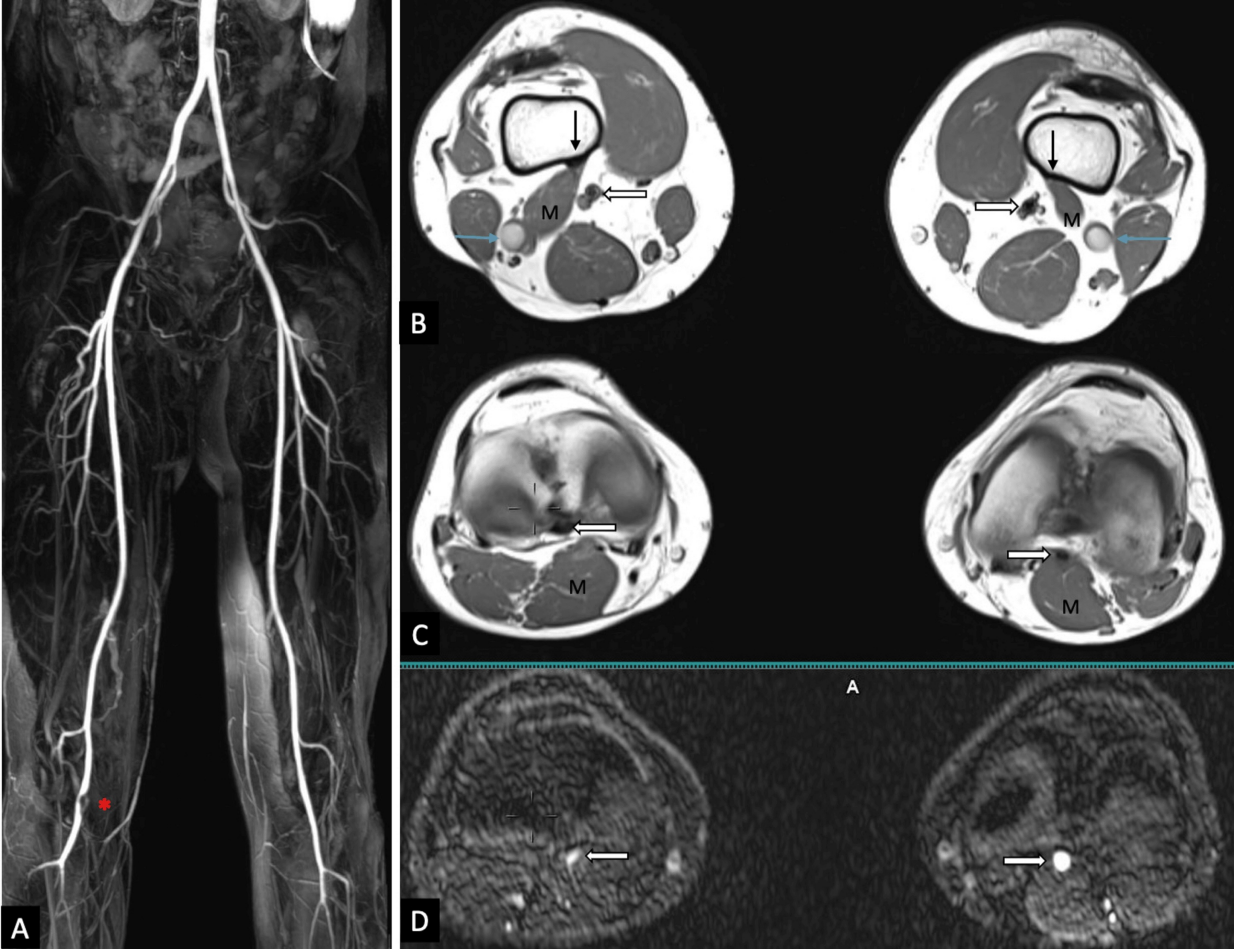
MRI of the popliteal region and non-contrast bilateral lower limb MR angiography of a patient with suspected PAES. Figure 2A's coronal MIP image of non-contrast MR angiography images shows an eccentric filling defect (red asterisk) involving the P2 part of the PA. Axial T1-weighted images (Figure 2B-2C) show an abnormally high and lateral insertion of the medial head of the gastrocnemius (M) just above the lateral aspect of the femoral condyle bilaterally (straight black arrow). The muscle belly is seen laterally deviated between the PV (blue arrow) laterally and the PA (white arrow) medially, causing significant extrinsic compression of the PA on the right side (white arrow; Figure 2B-2D). No significant compression of the PA (white arrow) was seen on the left side (Figure 2B-2D). MRI: magnetic resonance imaging; PAES: popliteal artery entrapment syndrome; MIP: maximum intensity projection; PA: popliteal artery; PV: popliteal vein
